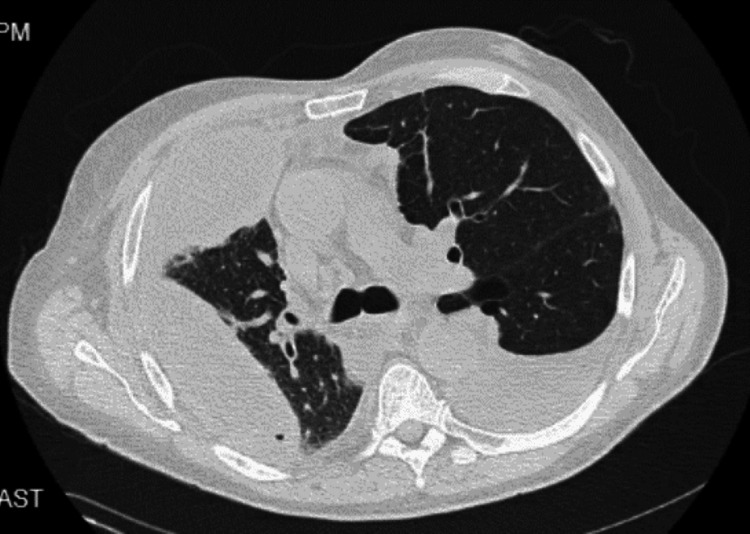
CT chest. Axial view (Contd.) Showing improved but persistent moderate loculated right pleural fluid with tiny foci of air with a chest drain within the inferior loculation
